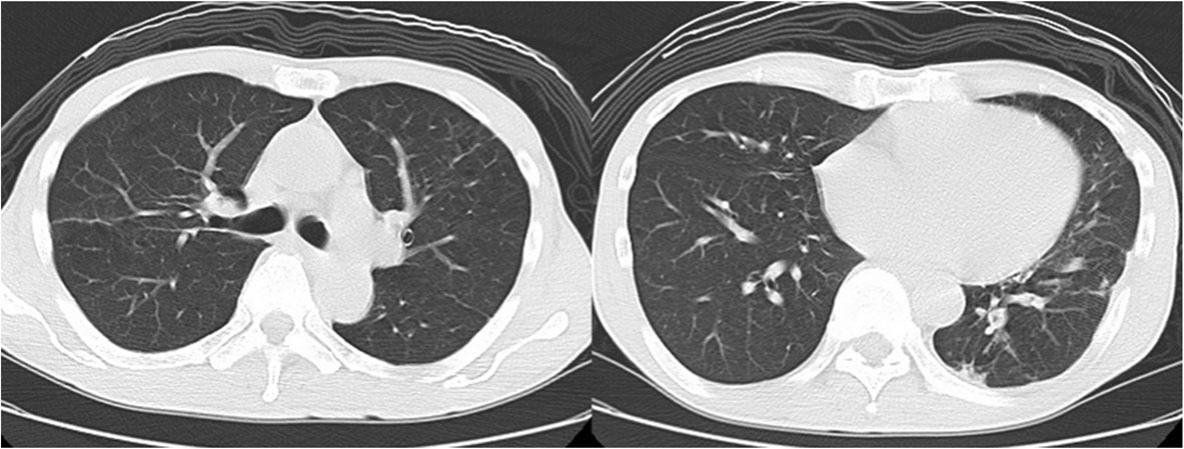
The chest CT scan after the completion of six-month antibiotic therapy showed a significant improvement
